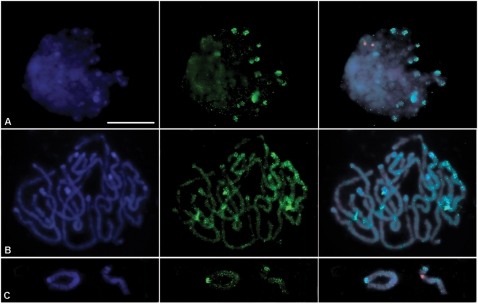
FISH on rye meristematic root-tip cells with REMAP product and pTa71. The following probes were used: total amplification product of REMAP reaction with primer combination Nikita/(CT)9G (green signal) and rDNA unit pTa71 (red signal). DNA was counterstained with DAPI (blue). (a) Interphase); (b) Prometaphase; and (c) Metaphase chromosomes. Bar 10 µm.
